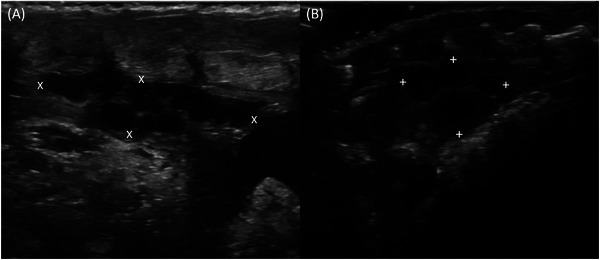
Parasagittal ultrasound images of ovaries, displayed with an 18 MHz linear transducer. Coupling from the dorsal side. A, ovary (X) of a bearded dragon. The previtellogenic follicles are anechogenic. B, ovary (+) of a leopard gecko, dorsally adjacent to the vertebral column and ventrally adjacent to the gastrointestinal tract.
